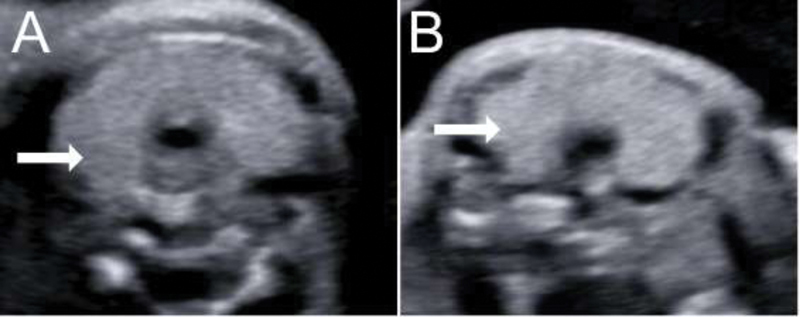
Two-dimensional ultrasonography of fetal thyroid gland (arrows) at the 28
